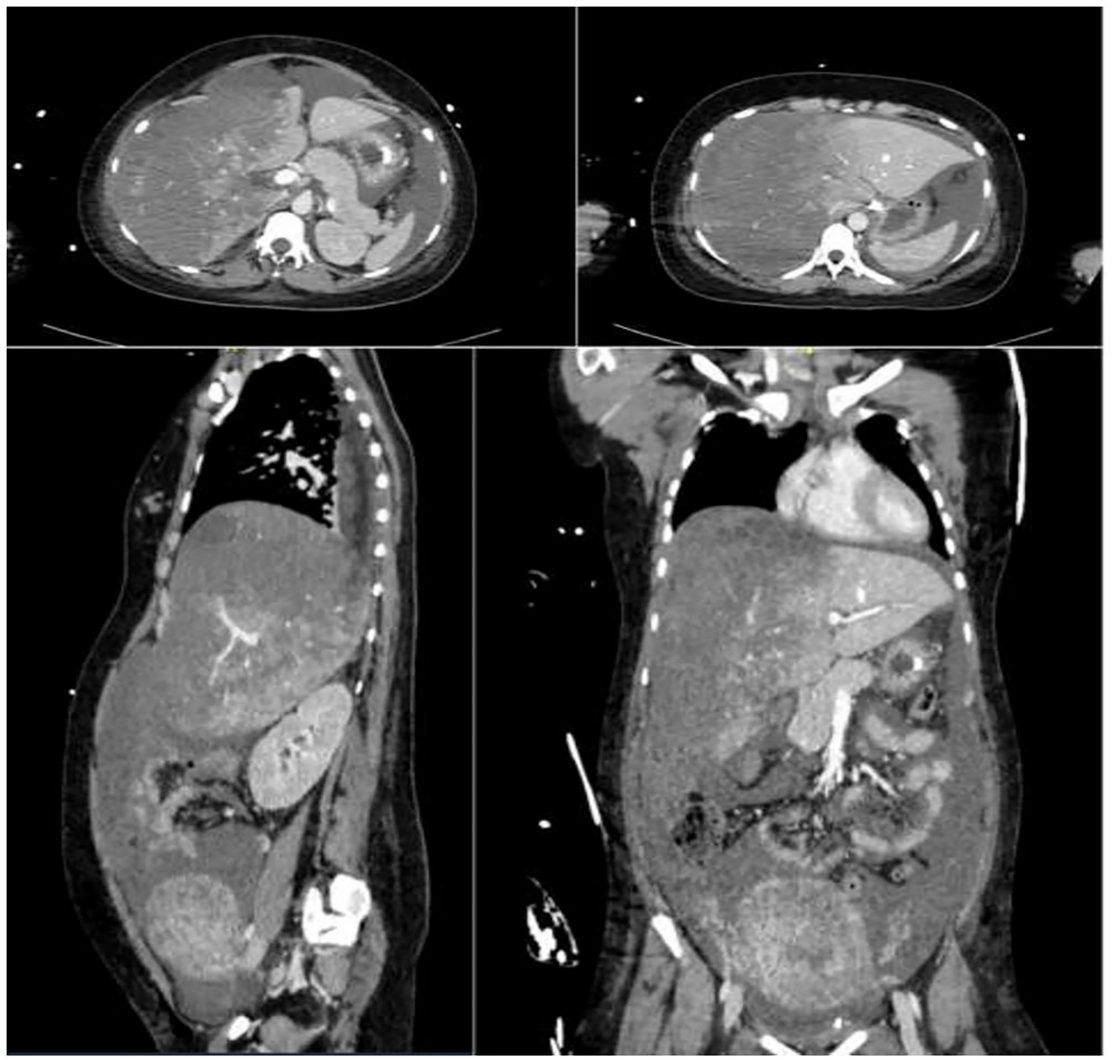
Computed tomography images of the large right liver haematoma with haemoperitoneum.
